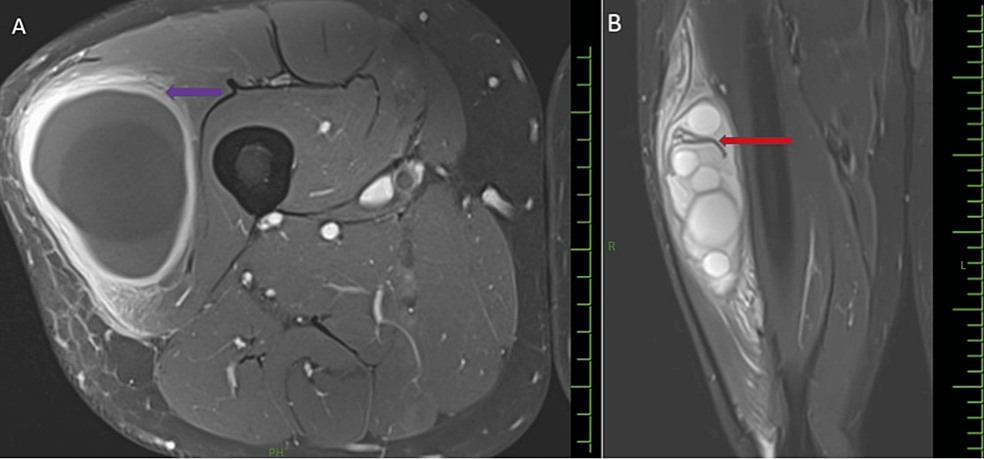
Gadolinium-enhanced MRI of the thigh: (A) axial showing the peripheral rim of enhancement (purple arrow); T2-weighted STIR coronal image (B) showing hypointense serpiginous foci within the cyst, consistent with the ruptured membrane (red arrow). MRI, magnetic resonance imaging; R, right; L, left; PH, posterior; STIR, Short Tau Inversion Recovery
